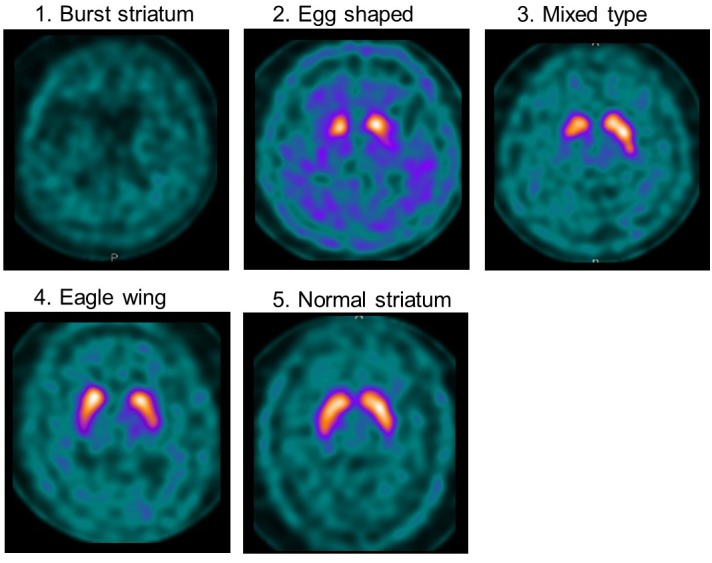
Examples of visual assessment using 123I-Ioflupane SPECT. Grade 1 (burst striatum): severe bilateral reduction with almost no uptake in both putamen and caudate nuclei or little uptake in the hemi-caudate nucleus with no uptake in the ipsilateral caudate nucleus. Striatal uptake of the same grade as background was judged as no uptake. Grade 2 (egg shaped type): bilateral reduction of tracer uptake with no uptake in putamen on either side and normal or almost normal uptake in caudate nuclei, resulting in an oval. Grade 3 (mixed type): normal or almost normal tracer uptake in bilateral caudate nuclei with asymmetrical tracer uptake in putamen. Grade 4 (eagle wing): normal bilateral uptake in caudate and almost normal and symmetrical uptake with a discrete reduction in one or both putamina, usually in lateral parts of the putamina and creating the shape of a wing. Grade 5 (normal striatum): symmetric tracer uptake bilaterally in putamen and caudate nuclei.
